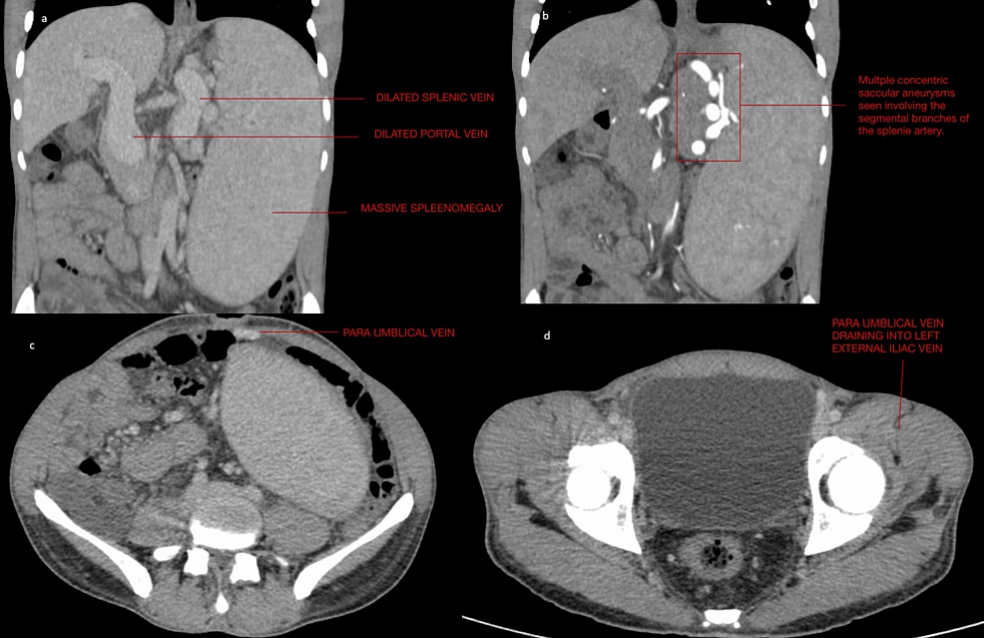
CECT scan showing (a) enlarged portal and splenic veins along with massive splenomegaly; (b) multiple aneurysms at the splenic artery; (c) dilated paraumbilical vein; and (d) paraumbilical vein draining into the left external iliac vein CECT, contrast-enhanced CT
